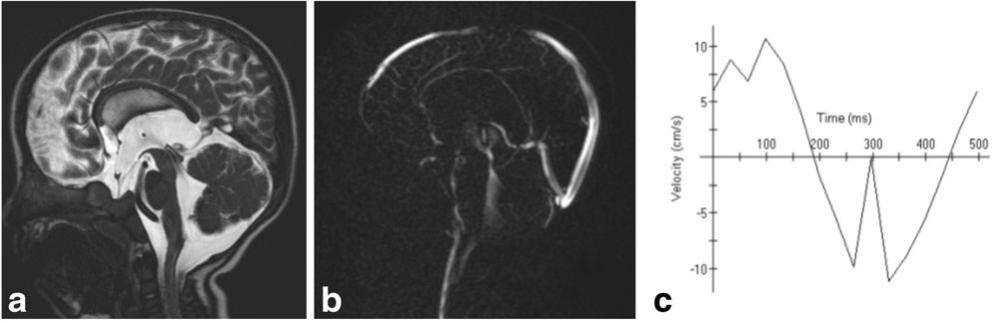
MRI of a 16-month-old boy with communicating hydrocephalus reveals hyperdynamic CSF flow through the aqueduct of Sylvius. a Sagittal 3D-DRIVE demonstrates flow void through the aqueduct of Sylvius. b Phase-contrast MRI of another patient demonstrates prominent CSF flow through the aqueduct of Sylvius with accelerated CSF flow velocity (c)
